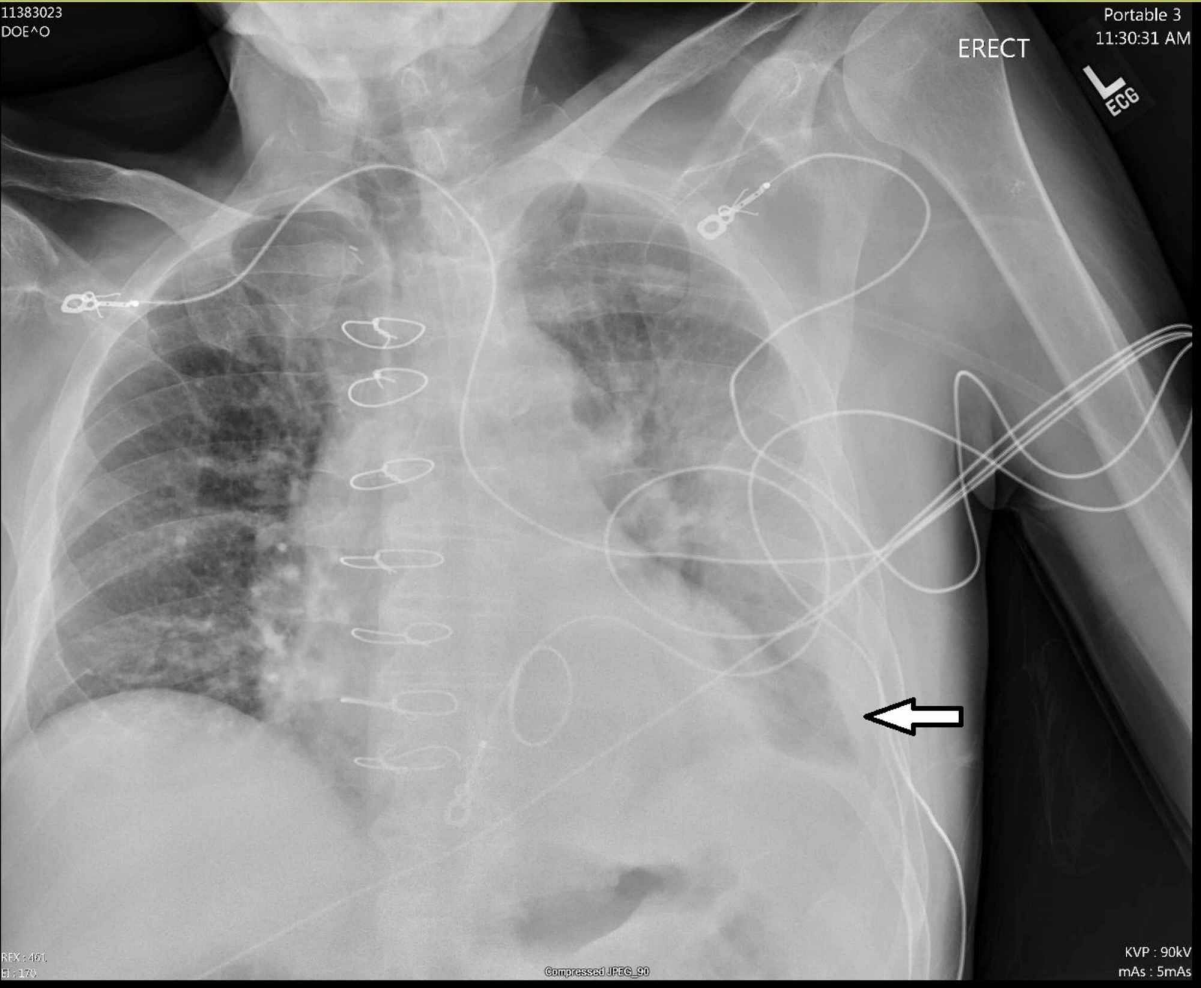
Chest X-Ray. Arrow pointing towards area of consolidation on left basilar region.
